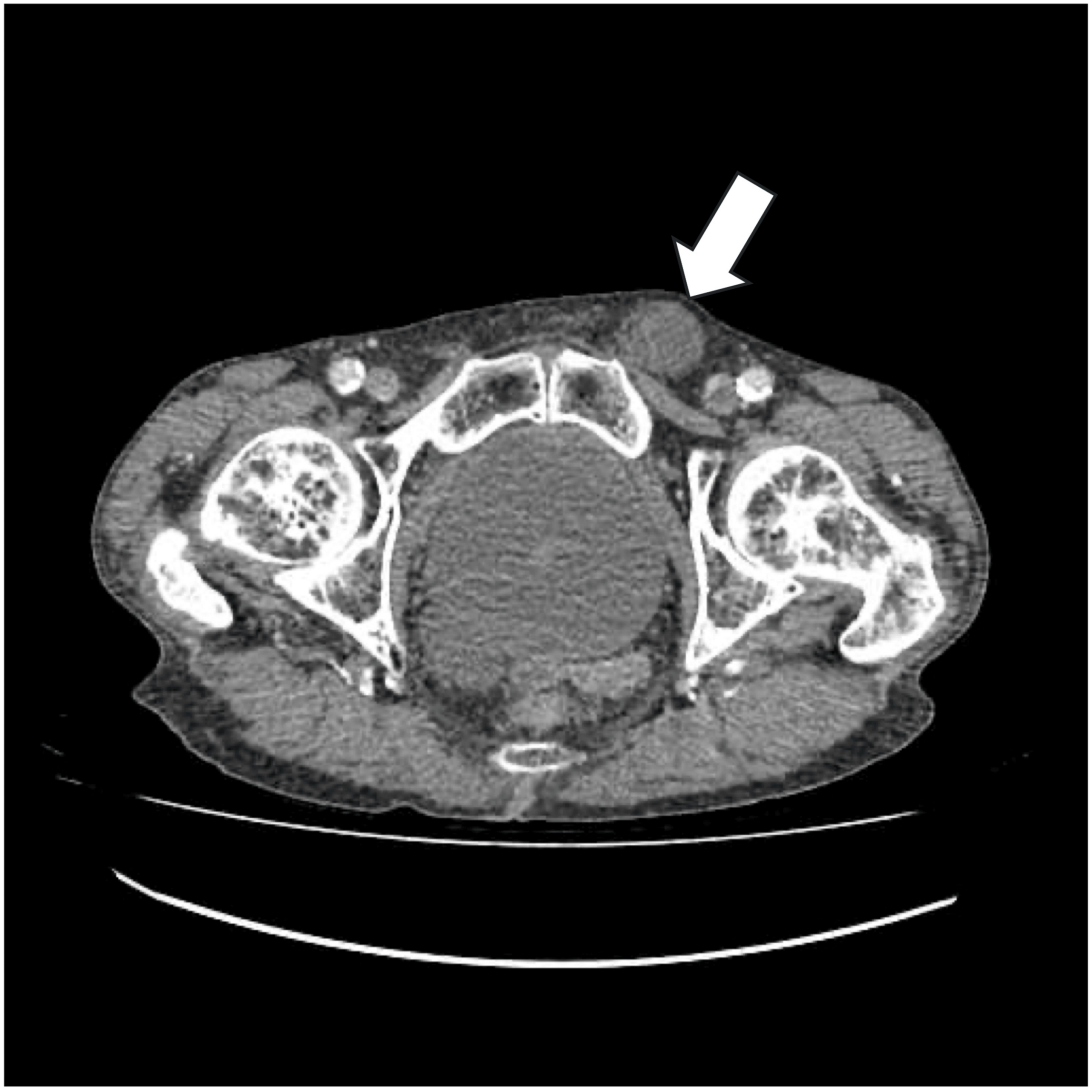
CT abdomen and pelvis showing strangulated left inguinal hernia (white arrow)
